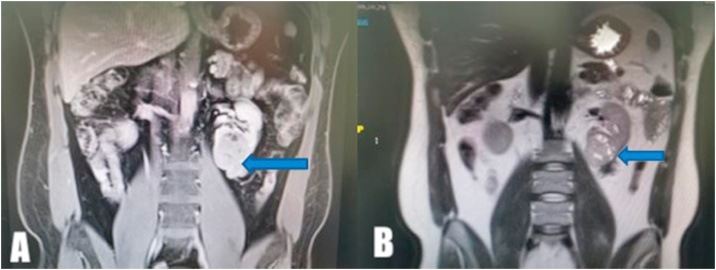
MRI, which showed left renal lower pole heterogenous mass measuring 6 × 5 × 4 cm suspicious for renal cell carcinoma.
